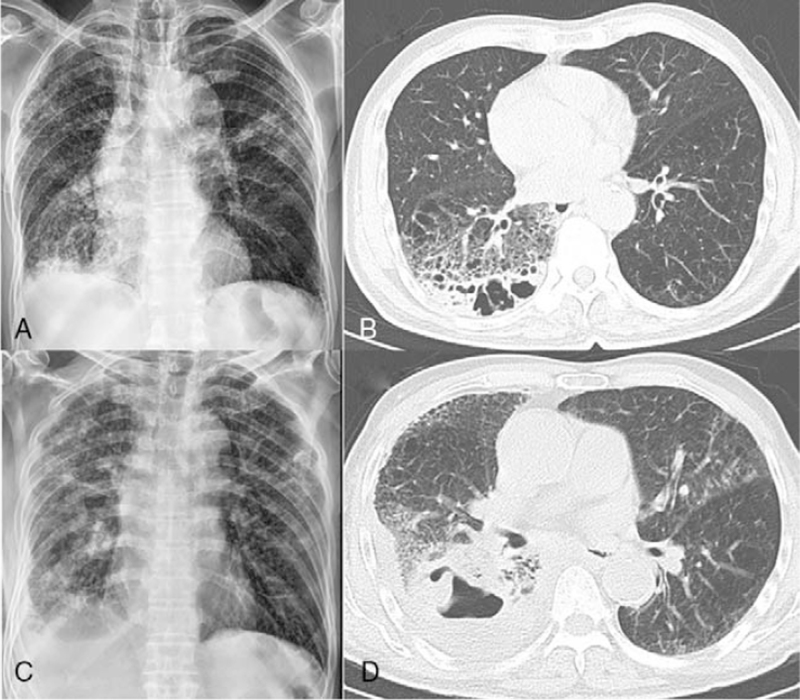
Chest radiographs and computed tomography (CT) images of case 2. (A) and (B) Images obtained 5 months before presentation. (C) and (D) Images obtained at presentation to the emergency room. (A) Chest radiograph showing consolidations in the right lower lobe. (B) Chest CT images showing a subpleural cavitary lesion with perilesional consolidation in the right lower lobe. (C) Chest radiograph showing a pleural effusion with consolidation in the right hemithorax. (D) Chest CT image revealing an increased subpleural cavitary lesion and pleural effusion in the right hemithorax.
